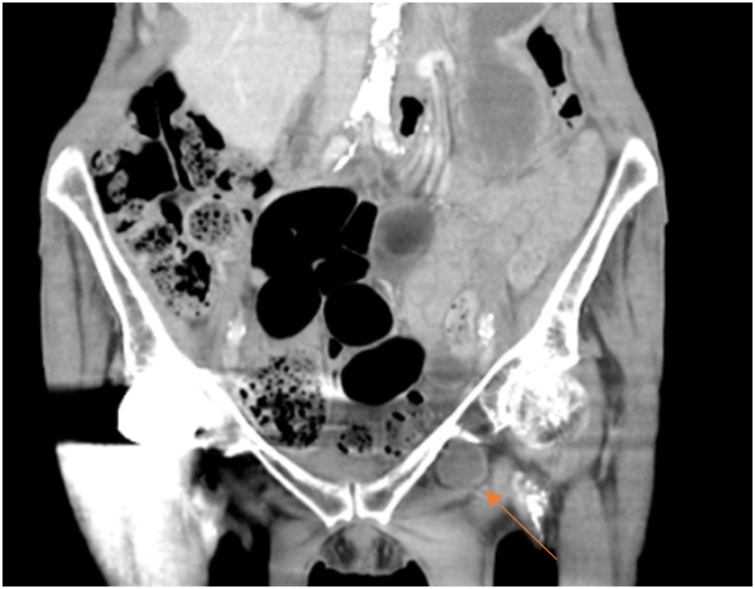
CT scan demonstration of a left obturator hernia.
